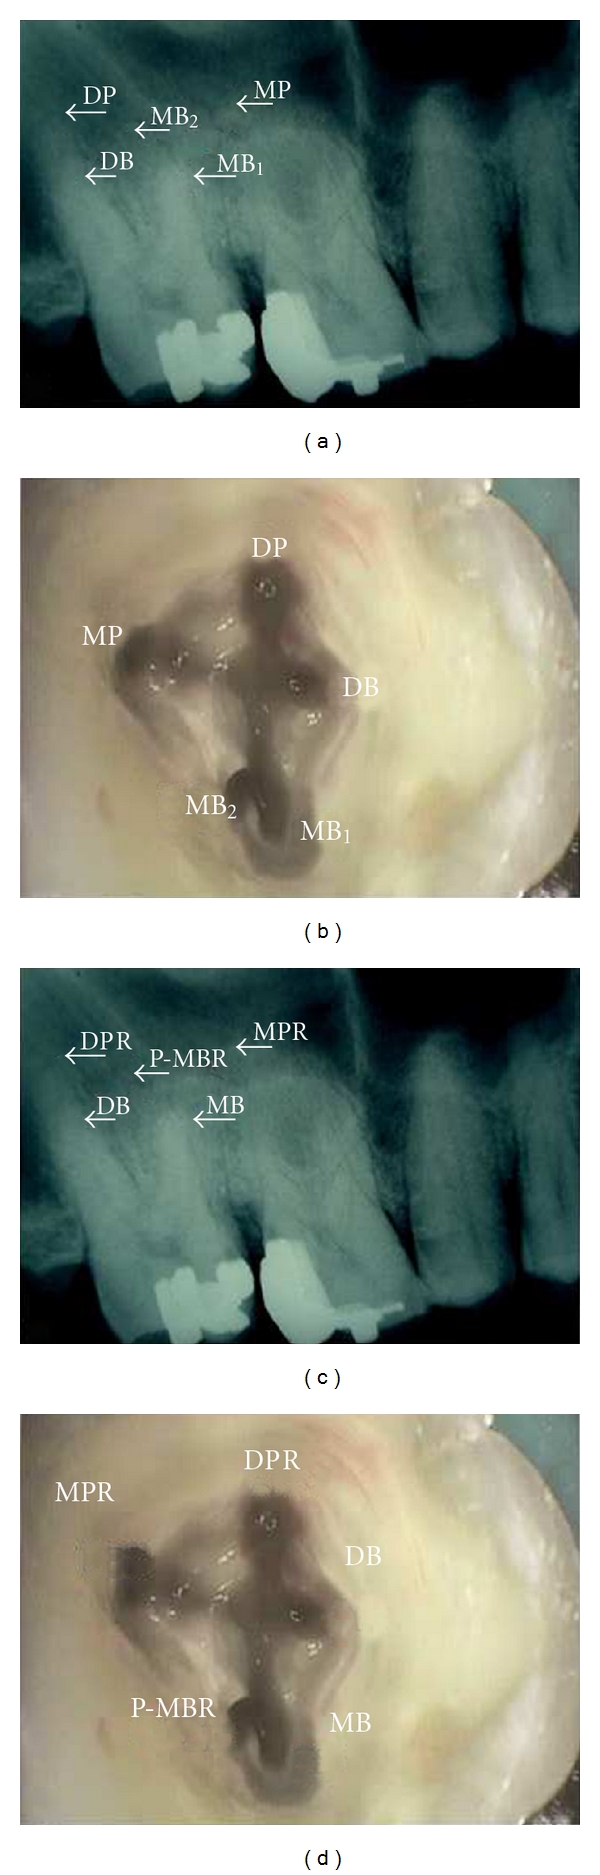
(a) Radiograph showing the root morphology of five separate roots named using the traditional terminology. MB: mesiobuccal, DB: distobuccal, DP: distopalatal, and MP: mesiopalatal. (b) Access opening picture of the same tooth showing the names of the canals as per the traditional approach, wherein each root has a single canal. (c) Radiograph, as seen in (a), with designation of roots according to their anatomical positions as per the proposed nomenclature. “*R*” signifies the presence of an additional root. *P-MBR*: palato-mesiobuccal root, *MPR*: mesiopalatal root, and *DPR*: distopalatal root. (d) Picture, as seen in (b), with naming of canals according to the recommended nomenclature. (Reprinted with permission from Kottoor et al. [4].)
